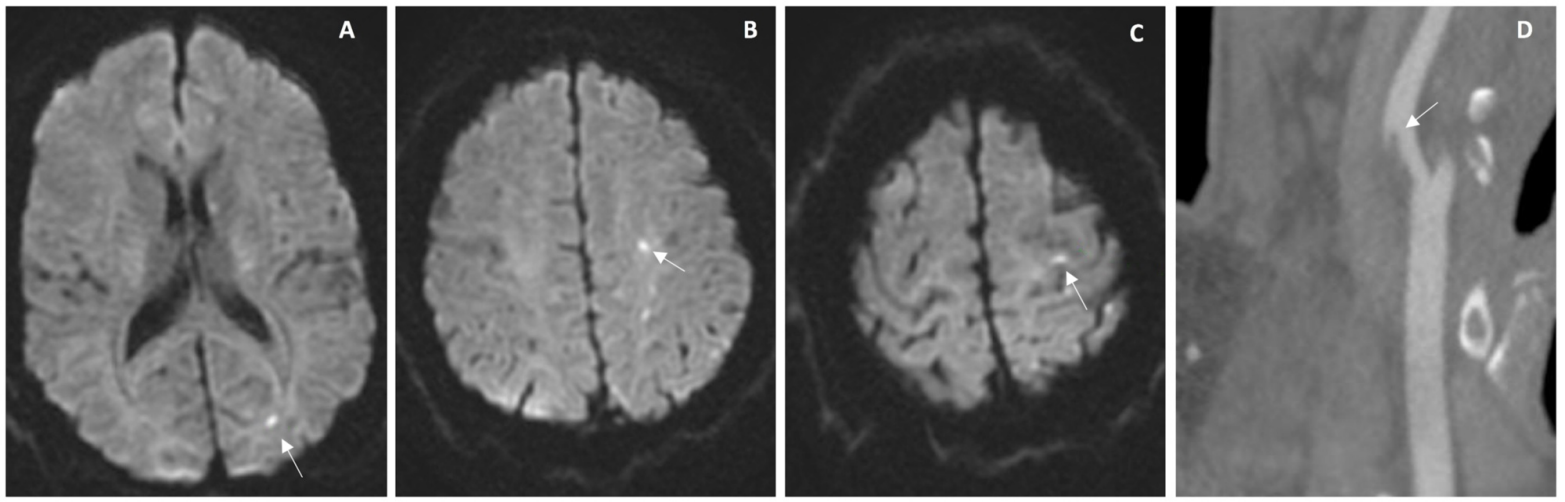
Imaging studies performed during hospital stay of case 2 Studies performed 48 hours after admission were MRI showing acute punctual infarctions (A-C) and computed tomography angiography demonstrating no change in the baseline degree of stenosis or intraluminal thrombus resolution (D)
